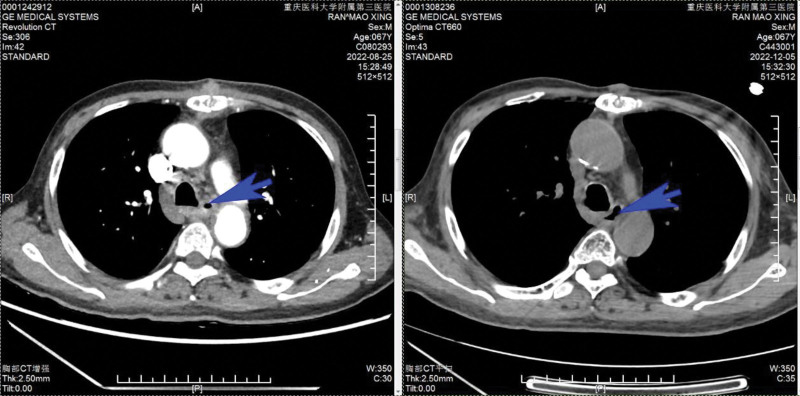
In this picture, the area indicated by the arrow is the esophagus. The left one is the CT on August 25th and the right one is the CT on December 5th. We can find that the thickening of the middle and lower esophageal wall is significantly reduced compared to before, and the stricture of esophagus is relieved.
